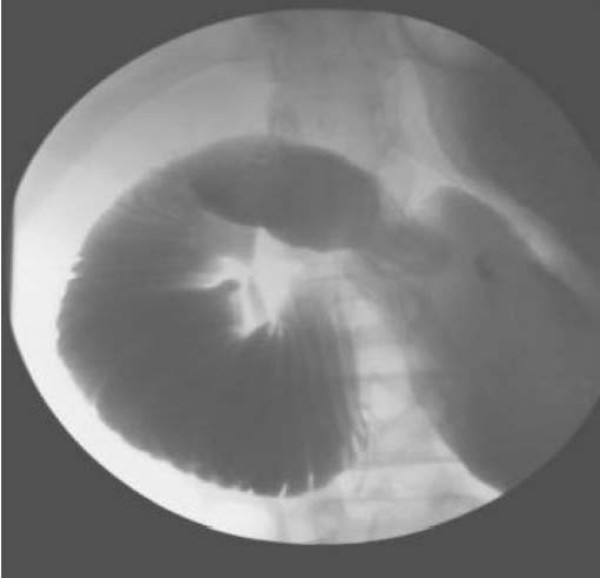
Barium swallow X-ray with small bowel follow-through demonstrating obstructed flow of contrast at the mid-third portion of the duodenum.
